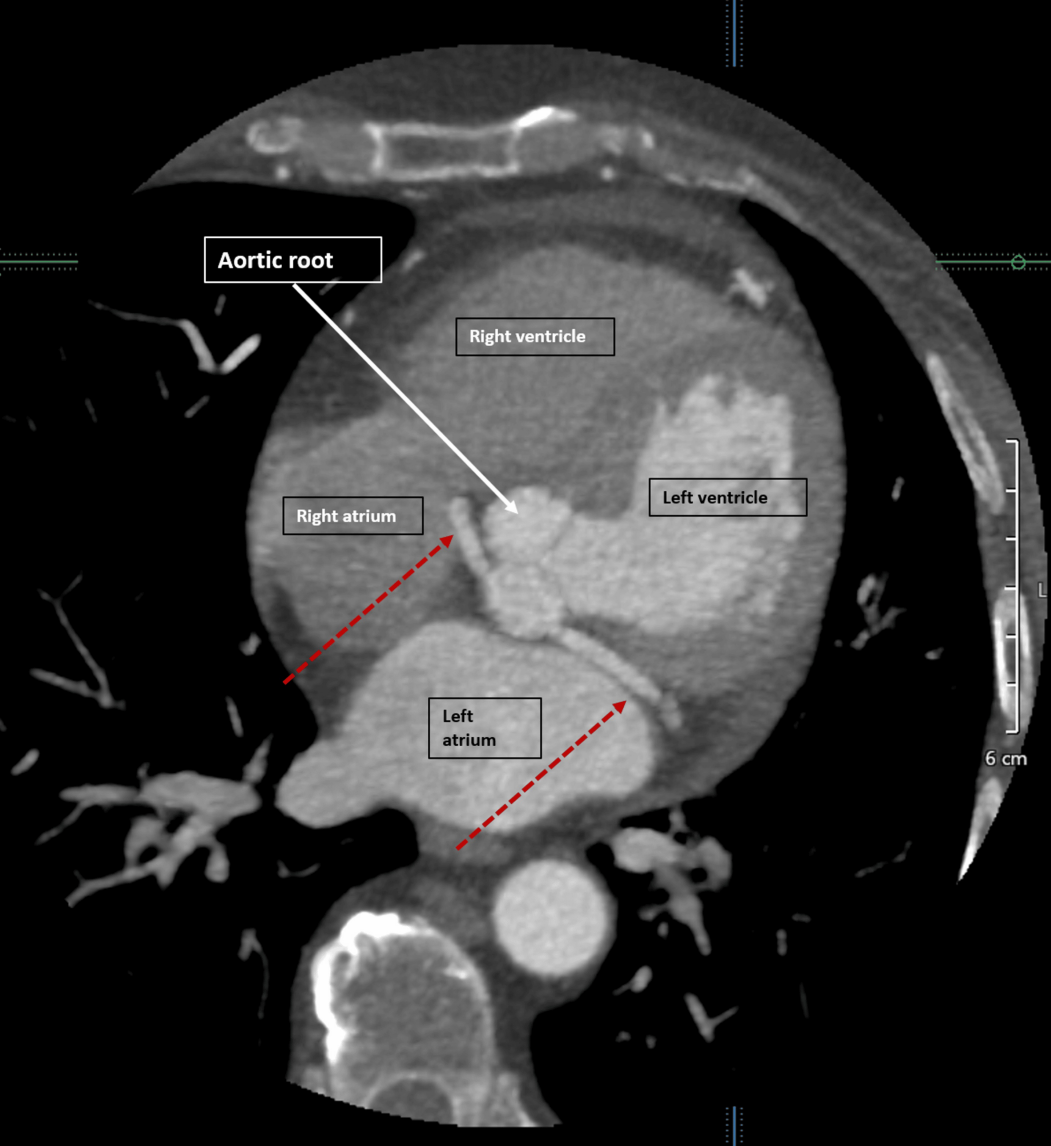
Coronary CT angiography (axial view) showing anomalous left circumflex coronary artery with a retro-aortic course to the left atrioventricular groove (red dashed arrows).
